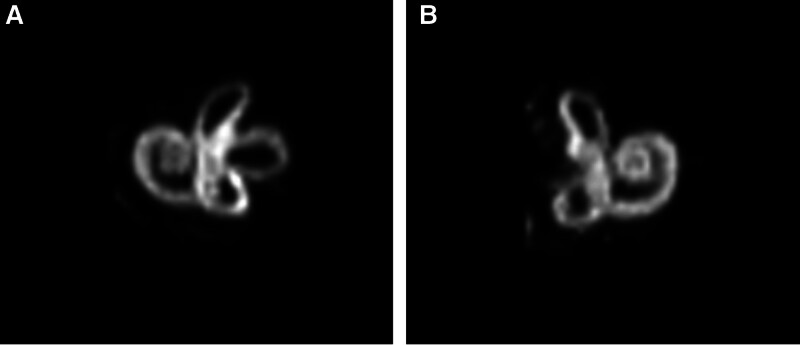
Three-dimensional magnetic resonance imaging (T2W_TSE) of the membranous labyrinth after horizontal semicircular canal occlusion. (A) Healthy ear. (B) Surgical ear. T2W_TSE = T2-weighted turbo spin echo.
